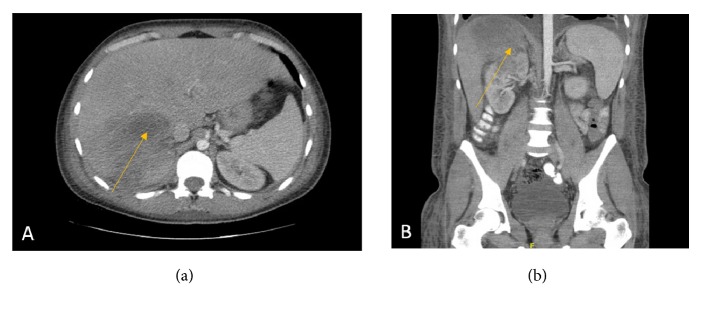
(a) Axial CT image of the abdomen showing a large multiloculated right hepatic mass extending from segment VIII to segment VI (arrow). (b) Coronal image in the same patient showing extension of the hepatic mass into the upper pole of the right kidney.
